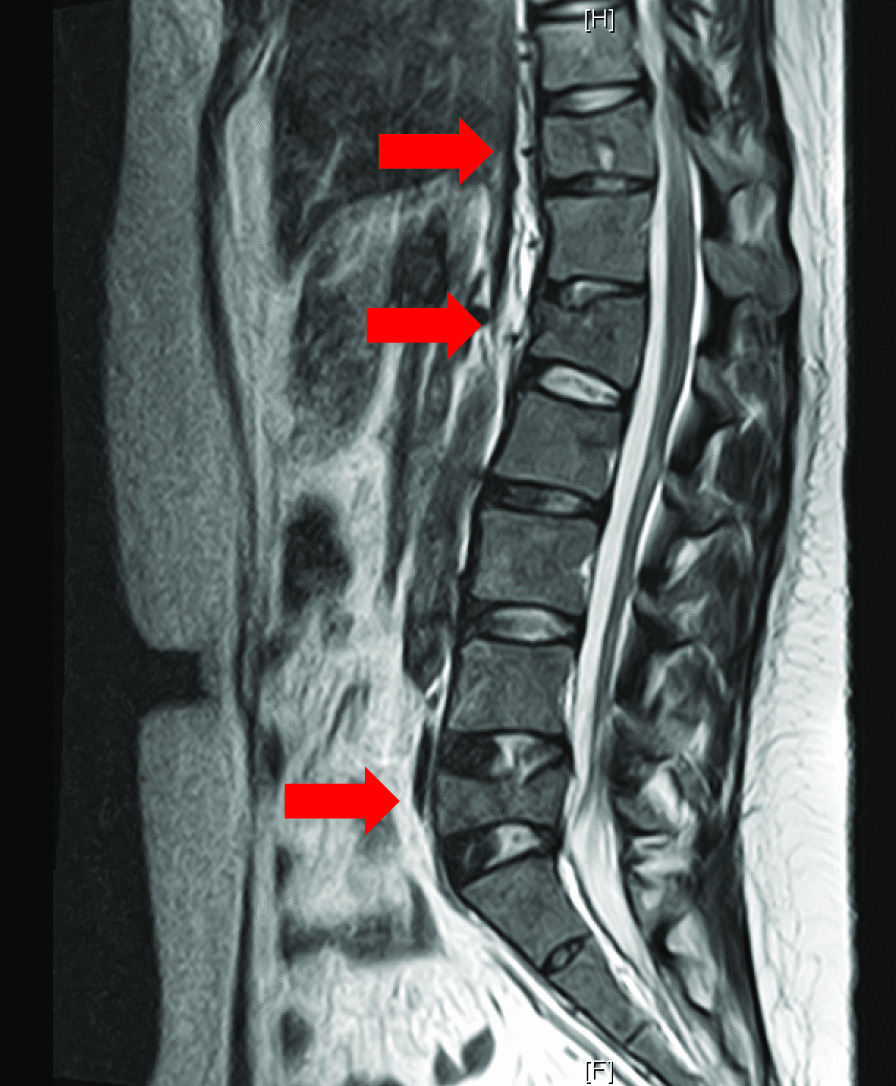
Thoracolumbar MRI T2 image. Multiple compression fractures at Th11, L1 and L5 (arrows)
